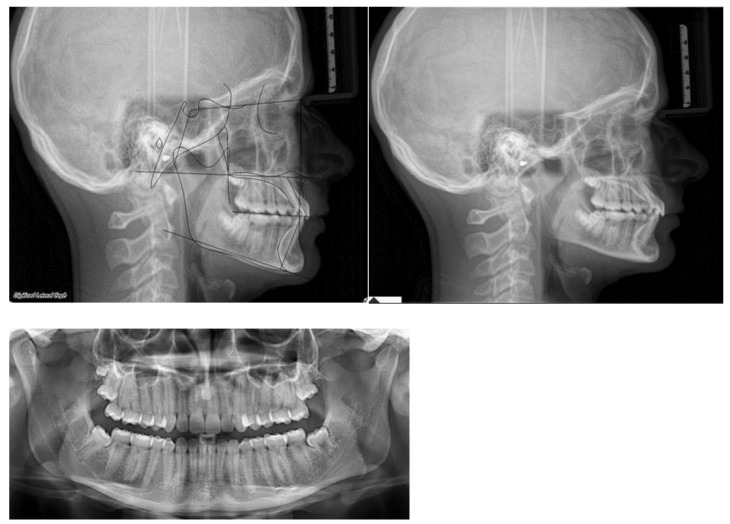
Post-treatment intraoral, extraoral photographs and radiographic examination.
